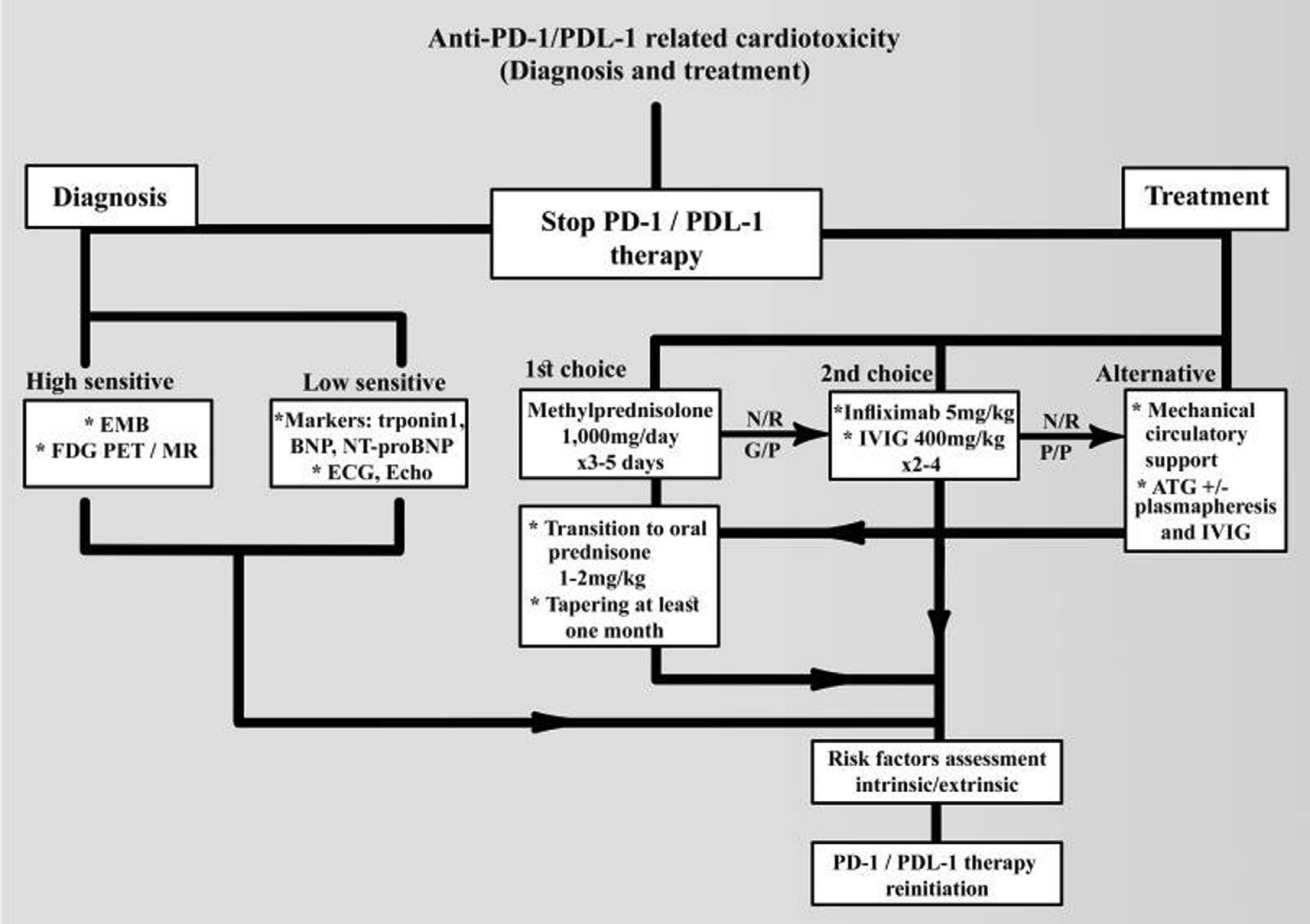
Diagnostic and treatment outlines for PD-1/PDL-1 drugs-related cardiotoxicity complications. FDG/PET: fluorodeoxyglucose/positron emission tomography, EMB: endomyocardial biopsy, BNP: brain natriuretic peptide, NT-proBNP: N-terminal pro-brain natriuretic peptide - N/R: no response. G/P: good performance. P/P: poor performance.
